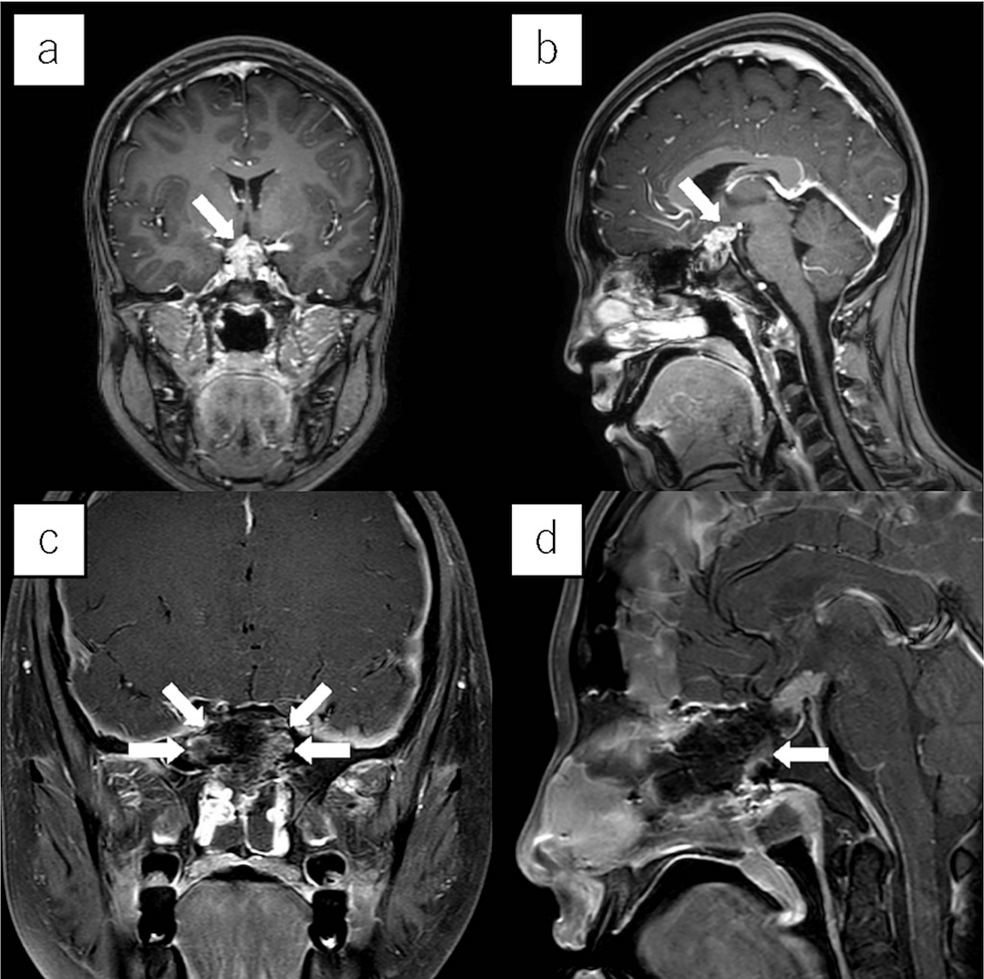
Pre- and postoperative contrast-enhanced MRI findings (a) Coronal and (b) sagittal contrast-enhanced magnetic resonance images before the fourth operation showed an enhancing 19 × 17 × 16 mm lesion within the sella that extended superiorly, dorsally, and to the right. The optic chiasm was mildly compressed anteriorly and superiorly. Tumor extension into the left cavernous sinus was suspected. The third ventricle was mildly compressed (white arrow). (c) Coronal and (d) sagittal images on the day after surgery showed contrast enhancement in the posterior septal nasal floor flap (white arrow); however, enhancement in the central region was minimal.
